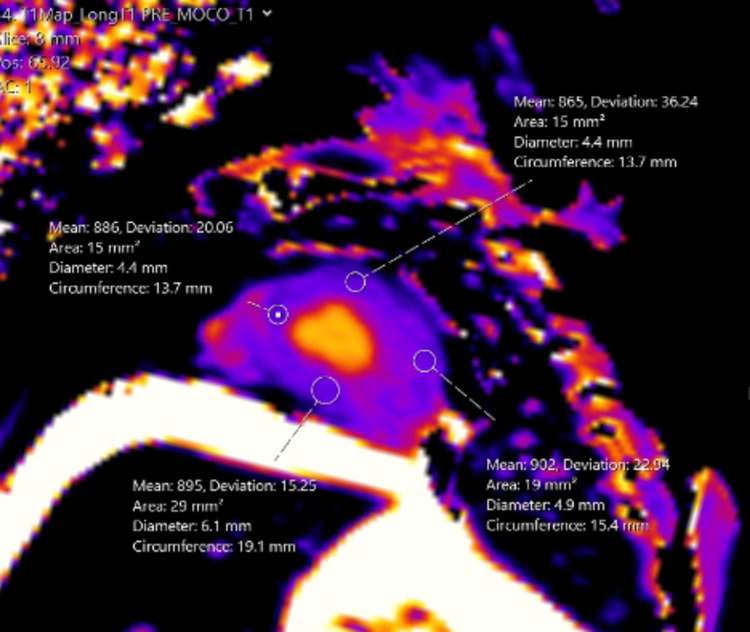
T1 parametric map demonstrating diffuse low T1 values corresponding to the diffuse hyperdensity seen on the CT.
